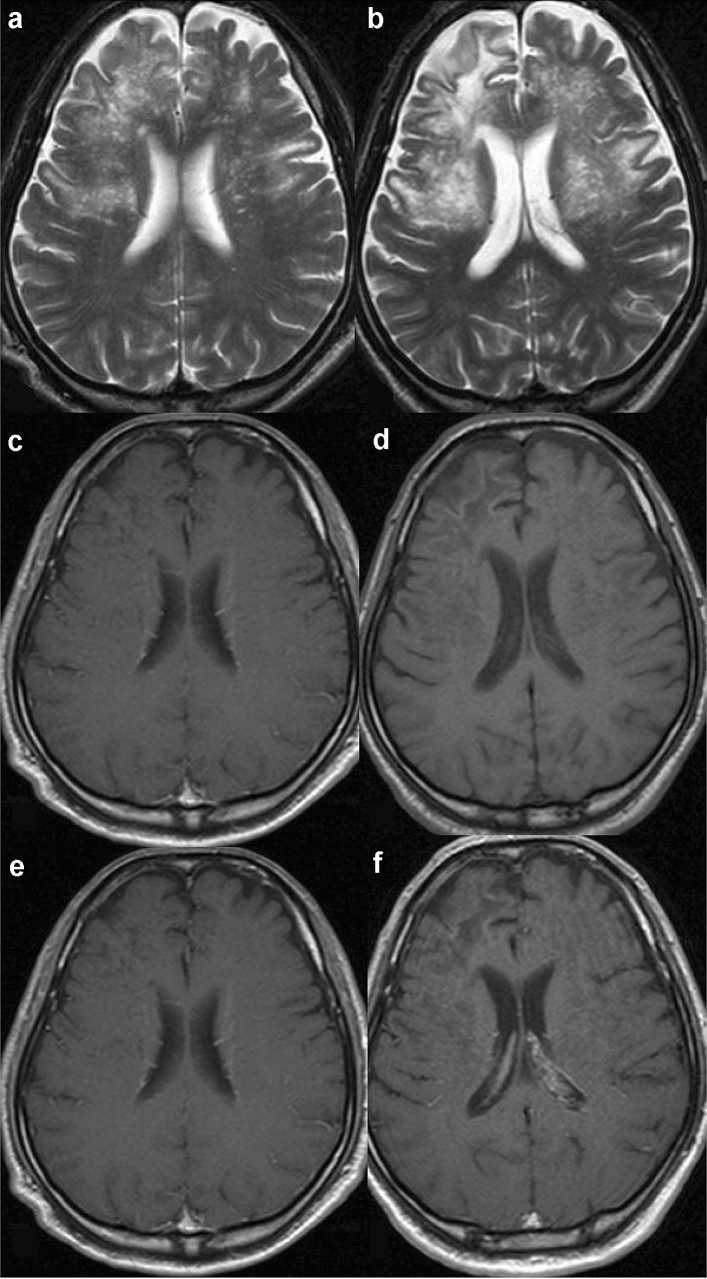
Lesion progression in a patient with HIV-related progressive multifocal leukoencephalopathy. T2-weighted (a, b), T1-weighted (c, d), and contrast-enhanced T1-weighted (e, f) images acquired 2 months (a, c, e) and 3 months (b, d, f) after the initial symptom of facial palsy. The first T2-weighted image (a) shows numerous dots with pale hyperintense lesions (milky way appearance) involving the bilateral frontal white matter predominantly on the right. The first T1-weighted image (c) shows hypointense lesions corresponding to advanced demyelination in right subcortical white matter. The second images (b, d, f) show more expansive lesions with more advanced demyelination than the first images (a, c, e). Milky way appearance is more prominent in the left frontal white matter on the second T2-weighted image (b) than on the first T2-weighted image (a)
